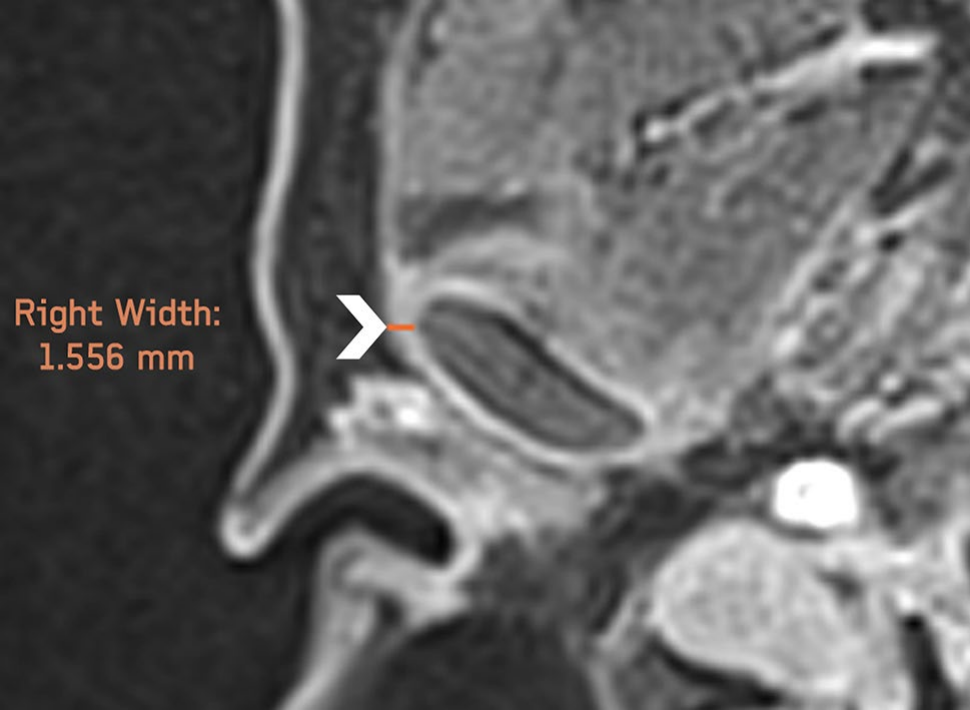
Axial postcontrast MRI image of the right temporomandibular joint. The lateral periarticular space width was measured from the most lateral point of the condylar cortex to the lateral limit of the periarticular tissue (arrowhead)
